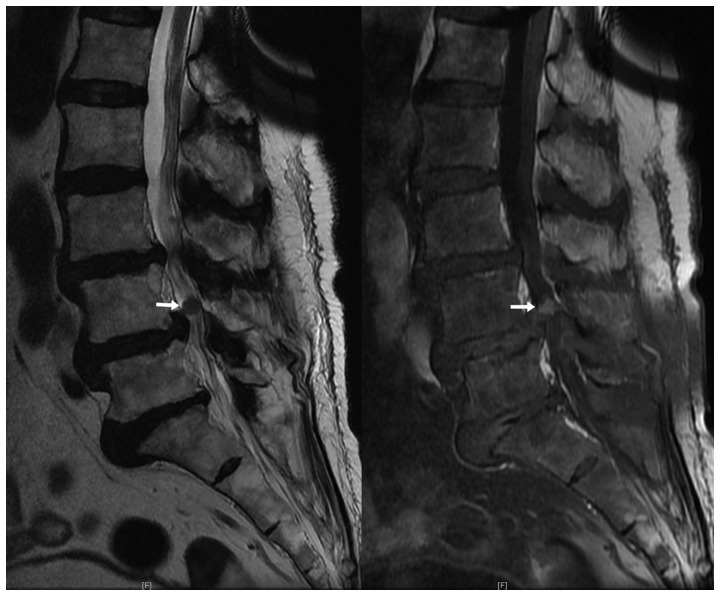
T2-weighted (left) and enhanced T1-weighted (right) magnetic resonance imaging revealing a well-rounded enhanced mass at the level of the L4–5 disc space, as shown by the white arrows.
